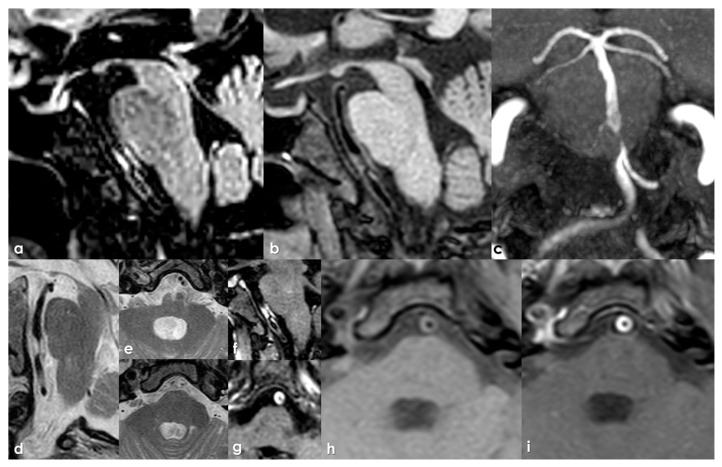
MRI and Magnetic Resonance Angiography (MRA) in the same patient referenced in Figure 1 and Figure 2. Panel (a,b): sagittal FLAIR at two different time points, respectively, with hyperintense BA walls corresponding with the plaque. Panel (c): MRA reconstructed in coronal plane with MIP/MPR protocol, confirming proximal BA stenosis, as in Figure 1 (CTA). Panel (d,e): sagittal and axial T2 Turbo Spin Echo (TSE) images at the level of the BA. Panel (f,g): corresponding T1 Dixon sagittal and axial images. Panels (h,i): magnification of proton density sequence pre- and post-contrast, respectively.
